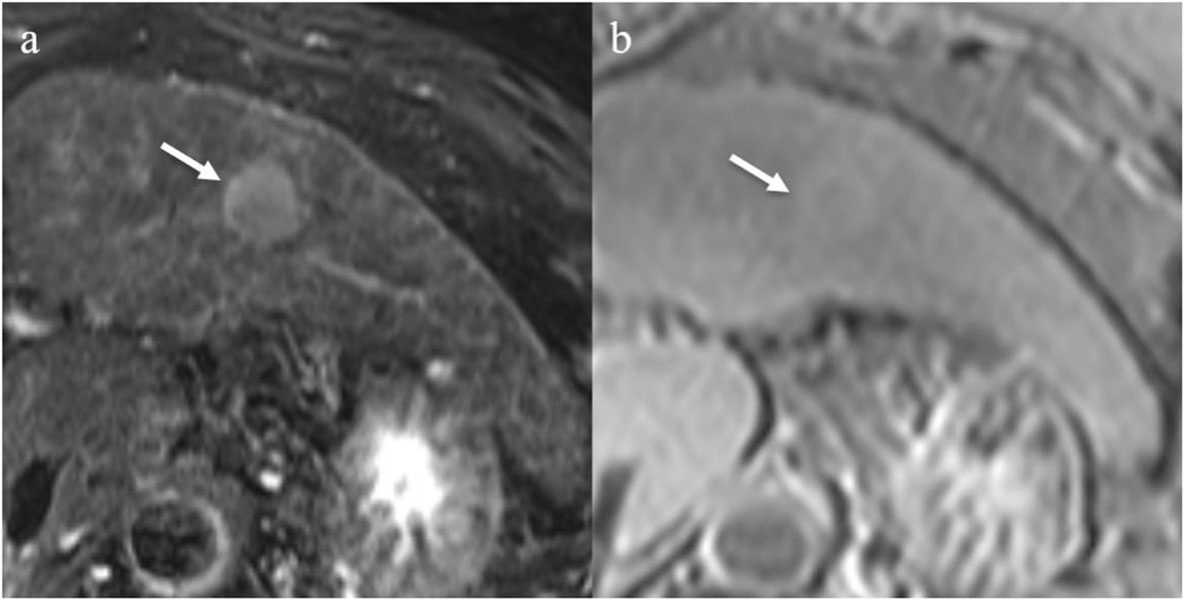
A 67-year-old female with hepatocellular carcinoma. a The tumor is seen as a hyperintense mass compared with the background liver on a T2-weighted imaging (white arrow). b The contrast between the tumor and the background liver is poor in the T2*-weighted image (white arrow)
